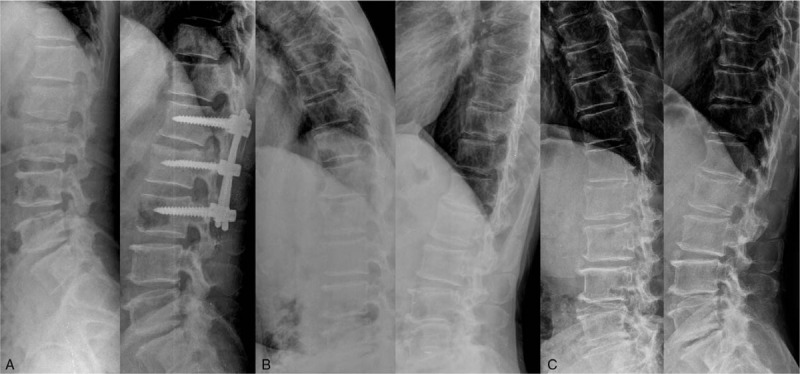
Lateral radiographs of a 51-year-old man with L1 burst fracture. Preoperative and immediate postoperative radiographs (A), flexion-extension lateral standing radiographs at 15 months (B), and 140 months after implant removal surgery (C). The segmental motion measured from flexion-extension lateral standing radiographs were 7.52 degrees (B) and 6.29 degrees (C), respectively.
